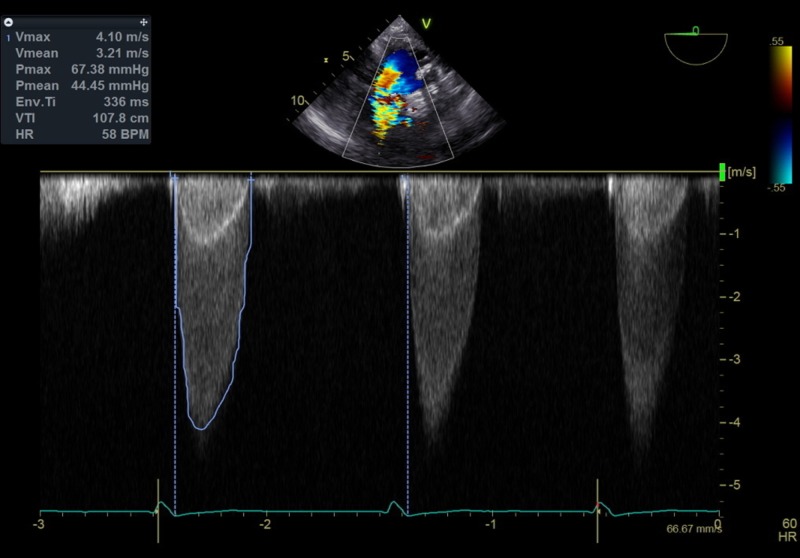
TEE image of continuous wave Doppler from deep transgastric view showing elevated velocities and gradients across the aortic valve TEE, transesophageal echocardiogram
